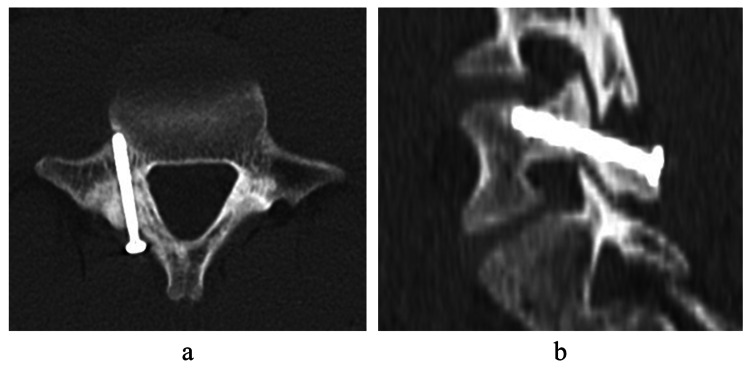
Postoperative radiographs at 4 months Computed tomography (CT) images 4 months after surgery. The lesion achieved bone union (a, b).
